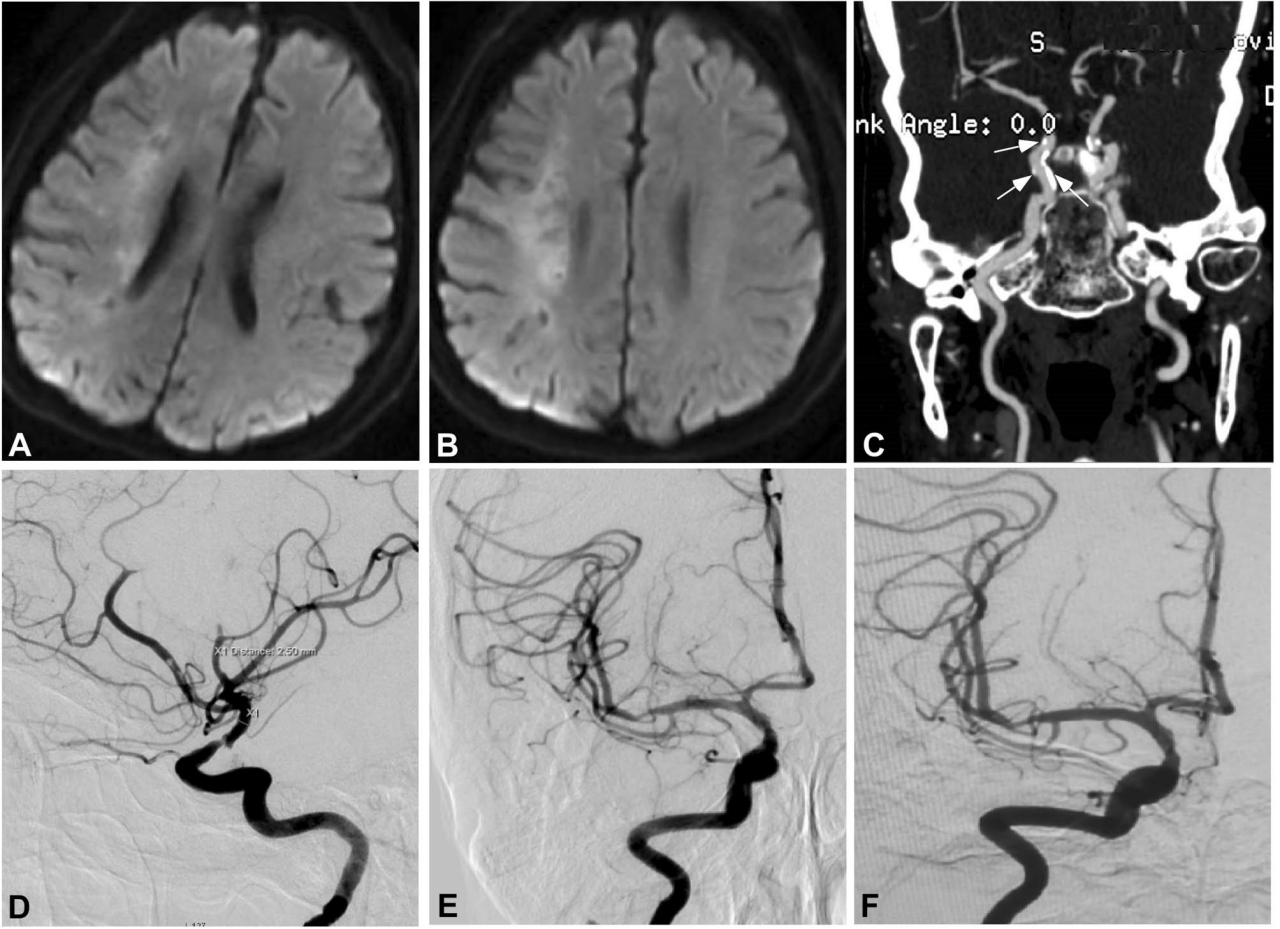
Right internal carotid artery severe stenosis. (A) Magnetic resonance imaging (MRI) showed an ischemic infarct at three days after onset. (B) MRI at 14 days after onset revealed increased area of the infarction. (C) Computed tomography revealed calcification (arrows) around the posterior communicating artery segment of the right internal carotid artery. (D) Cerebral digital subtraction angiography demonstrated severe stenosis at the posterior communicating artery segment of the right internal carotid artery. (E) Stent angioplasty was performed with an Enterprise stent and the severe stenosis disappeared. (F) Nine months after stent angioplasty, the stent remained patent.
